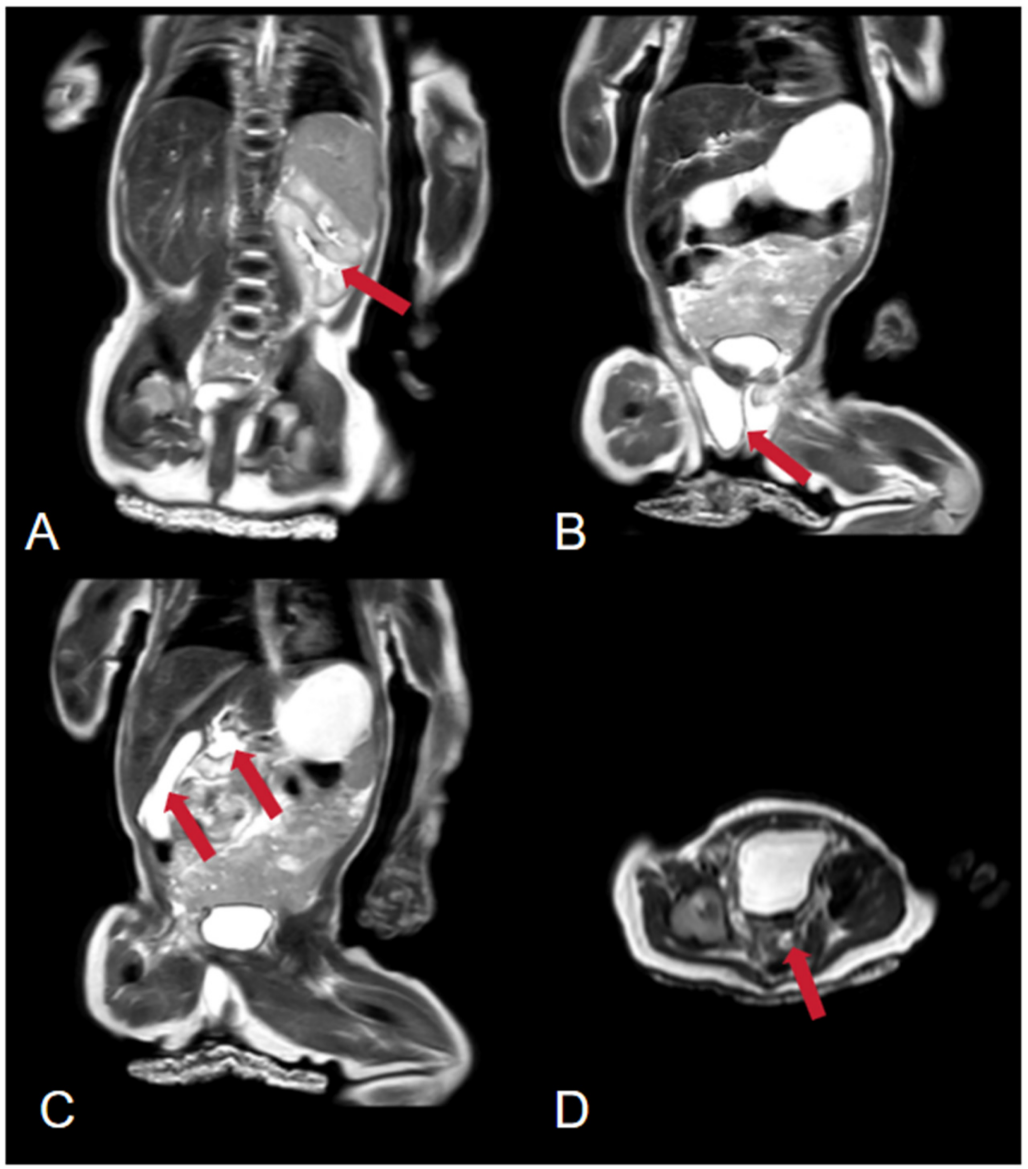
Abdominal magnetic resonance imaging (MRI). (A) The arrow points to a duplicated left kidney. (B) The arrow points to the testes. (C) The arrow points to the gallbladder and bile duct tissue. (D) The arrow points to a suspected uterine signal.
